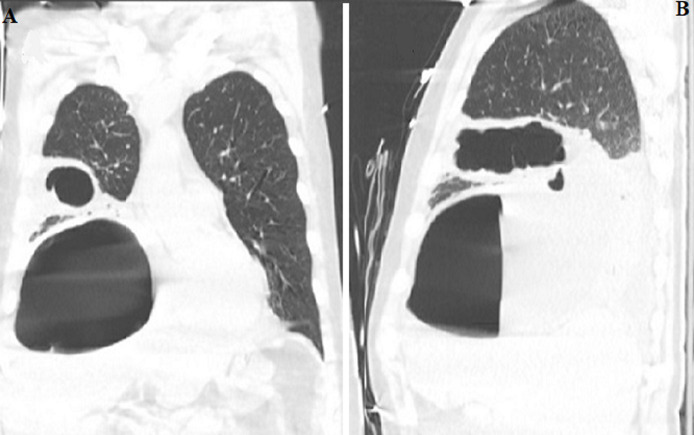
A) coronal chest CT on day 3 showing a significant increase in the size of the abscess cavity and dense consolidation in RLL; B) sagittal chest CT on day 3 showing a significant increase in the size of the abscess cavity and dense consolidation in RLL
